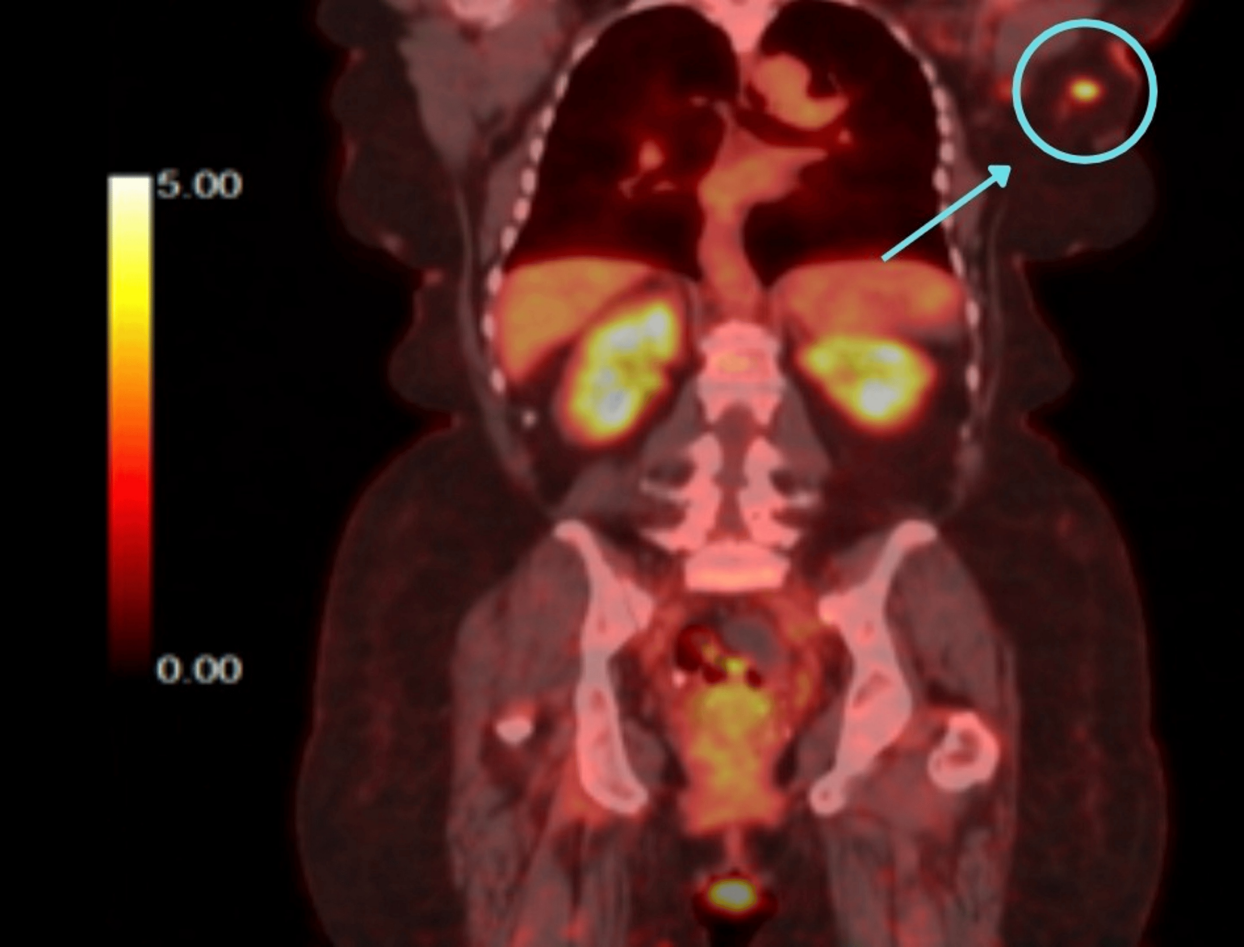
Coronal view of the PET/CT scan showing an FDG-avid left axillary node FDG: Fluorodeoxyglucose
